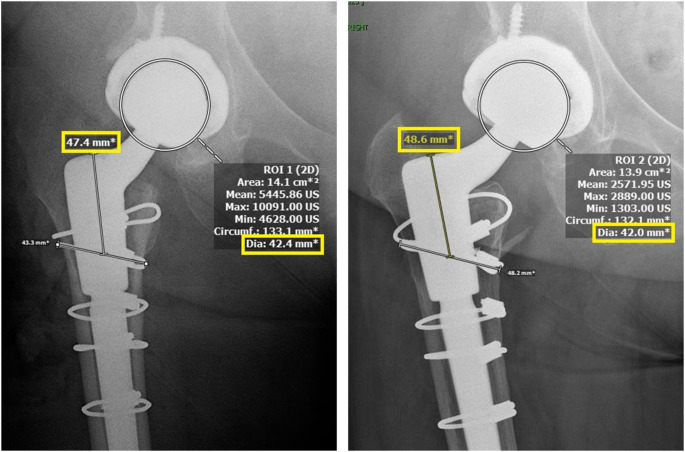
Example Subsidence Measurement. Intraoperative AP radiograph (left), *≥* 1-year postoperative radiograph (right)
